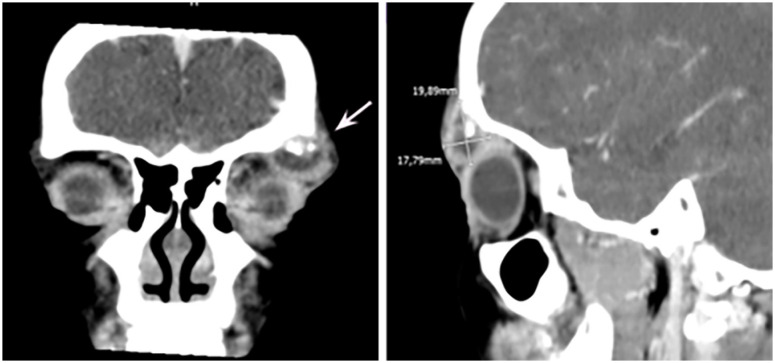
Cranial computed tomography showing an orbital abscess.
